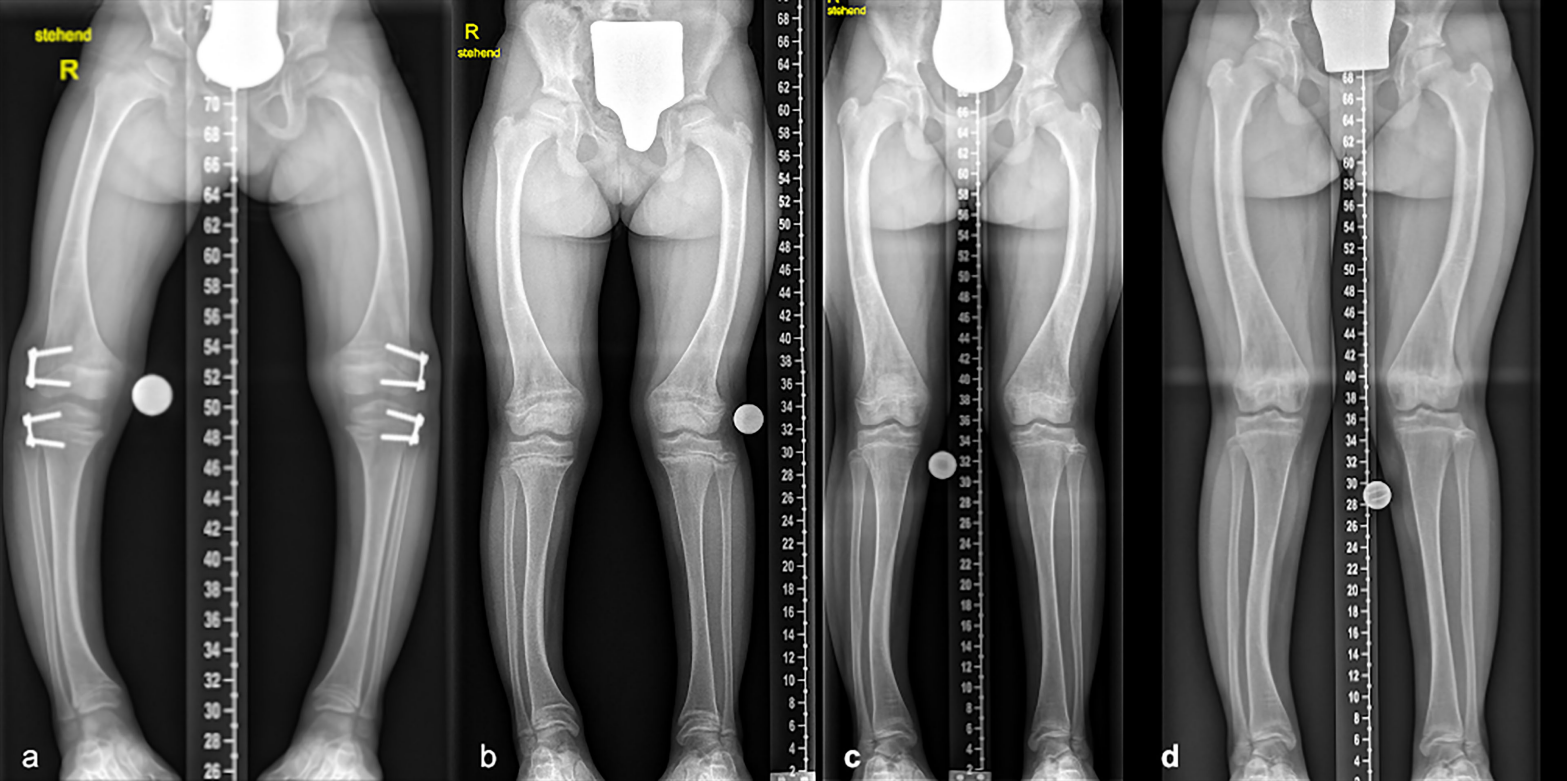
Radiographic progress of lower limb deformity in a 9 years 11 months old girl with familial XLH (case 6). Guided growth surgery was performed at age 5 years 1 month for varus deformity many years before Burosumab therapy (A). Mechanical axis remained stable during follow-up before initiation of treatment (B), at the one-year follow-up (C), as well as the last follow-up 6.5 years after guided growth (D).
